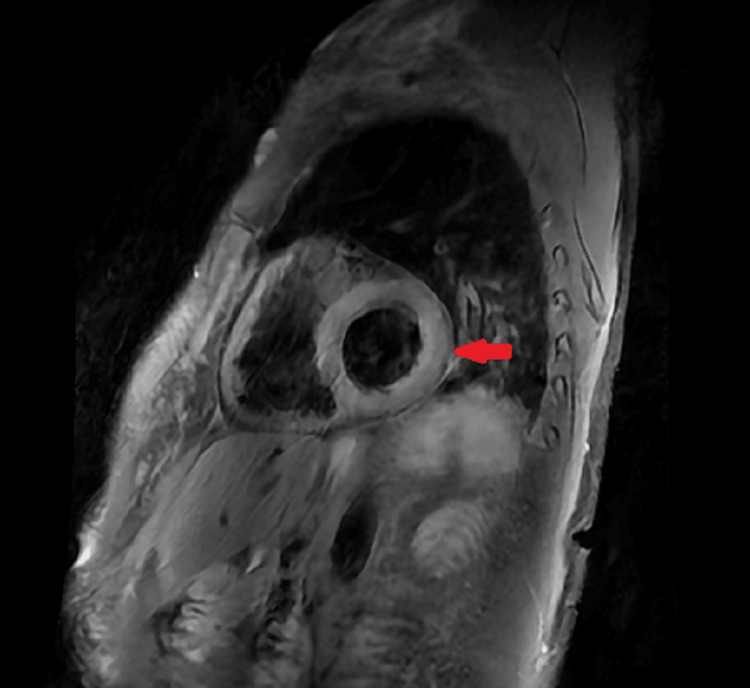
Cardiac MRI T2-weighted black blood image showed no increased signal in the cardiac wall (red arrow), suggesting no myocardial oedema.
